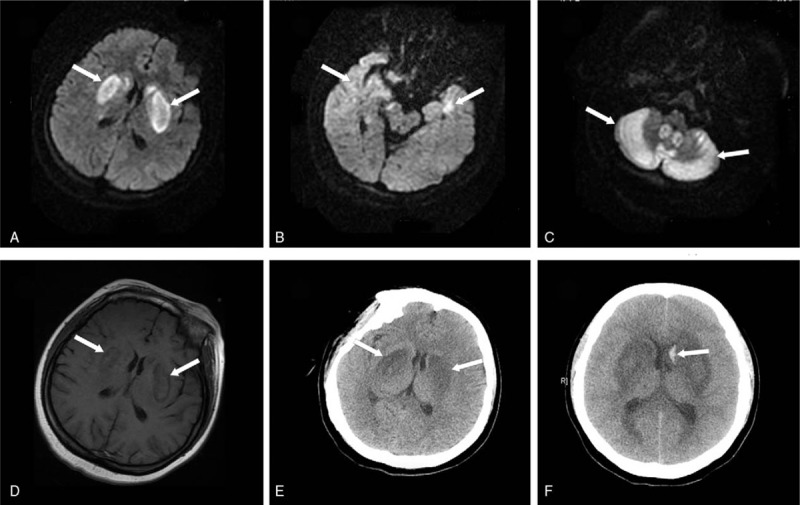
Brain magnetic resonance imaging and computed tomography (CT) scans. Diffusion-weighted imaging shows bilateral hyperintensity lesions of basal ganglia (A, arrows), temporal lobe (B, arrows), and cerebellum (C, arrows), with corresponding hypointensity lesions of basal ganglia on T1-weighted image (D, arrows). CT scan shows decreased attenuation of bilateral basal ganglia (E, arrows). And a repeat CT scan 3 days thereafter shows bleeding of the left caudate nucleus (F, arrow).
